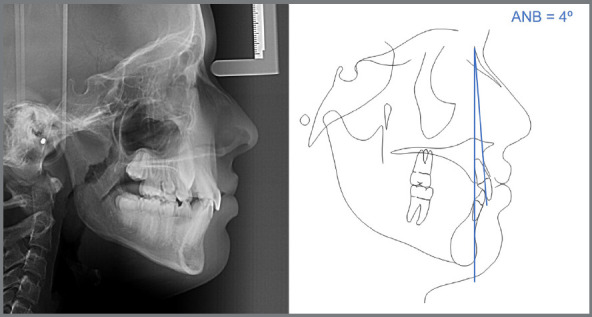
Initial radiograph and cephalometric tracing with measurement of the ANB angle.
